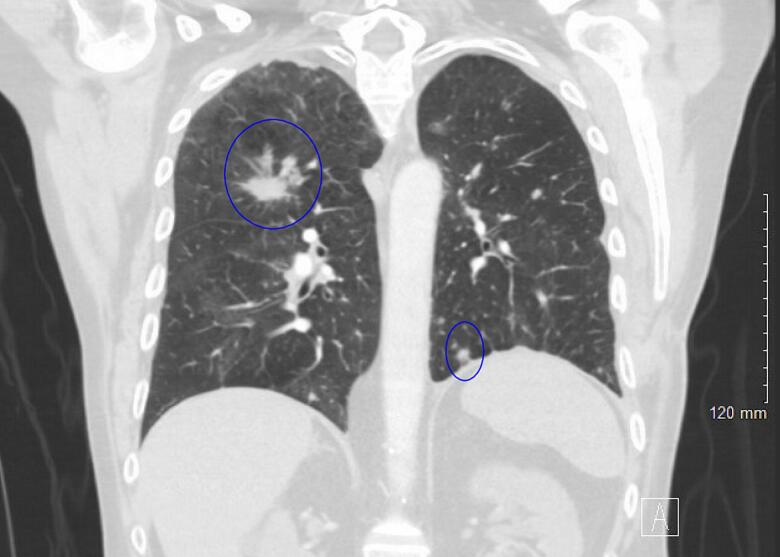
CT chest 2 months postoperatively with complete left lung expansion and stable bilateral nodules. (blue circles). (For interpretation of the references to colour in this figure legend, the reader is referred to the web version of this article.)
